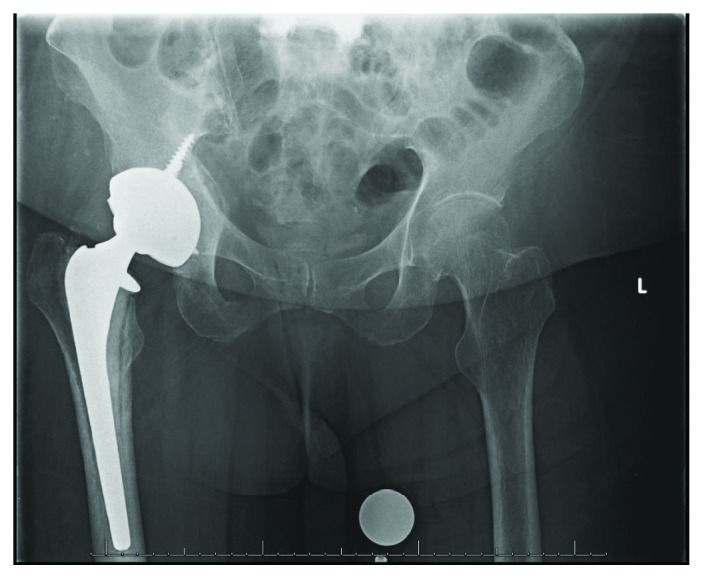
AP pelvis radiograph obtained 2 weeks after revision to total hip arthroplasty. No evidence of component loosening. Fracture line without displacement.
